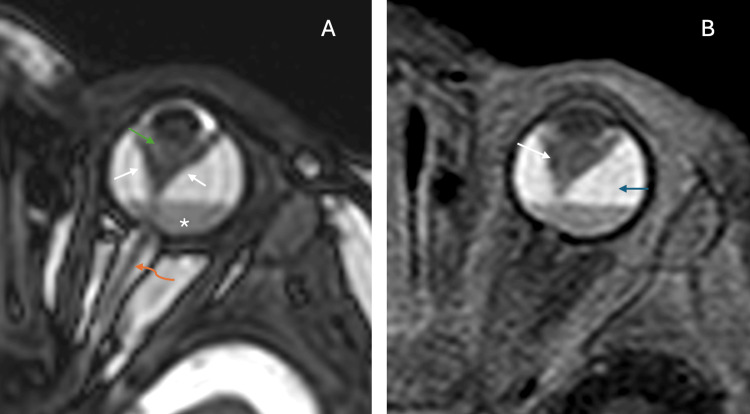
Axial magnetic resonance imaging of the orbits in a one-month-old infant with persistent hyperplastic primary vitreous (PHPV). (A) Axial heavily T2-weighted image (TR/TE 2500/120 ms, 3-mm slice thickness) demonstrates marked left microphthalmos with an abnormal funnel-shaped hypointense structure within the vitreous cavity. The detached retina forms a characteristic V-shaped configuration (white arrowheads) with its apex converging posteriorly at the optic disc. An ill-defined hypointense structure (green arrow) extends from the posterior lens surface to the optic nerve head, representing the persistent hyaloid artery within Cloquet's canal. Heterogeneous signal intensity within the vitreous cavity suggests hemorrhage with fluid-fluid levels (indicated by an asterisk). The lens appears dysplastic and has irregularity along the posterior margin. The left optic nerve is hypoplastic (curved orange arrow). (B) Axial post-contrast T1-weighted fat-saturated image (TR/TE 516/12 ms, 3-mm slice thickness) reveals enhancement of the retrolental fibrovascular tissue (white arrow) and the persistent hyaloid stalk. The subretinal space demonstrates intrinsic T1 hyperintensity (blue arrow) consistent with proteinaceous fluid or hemorrhage, without significant enhancement. No considerable enhancement noted elsewhere.
